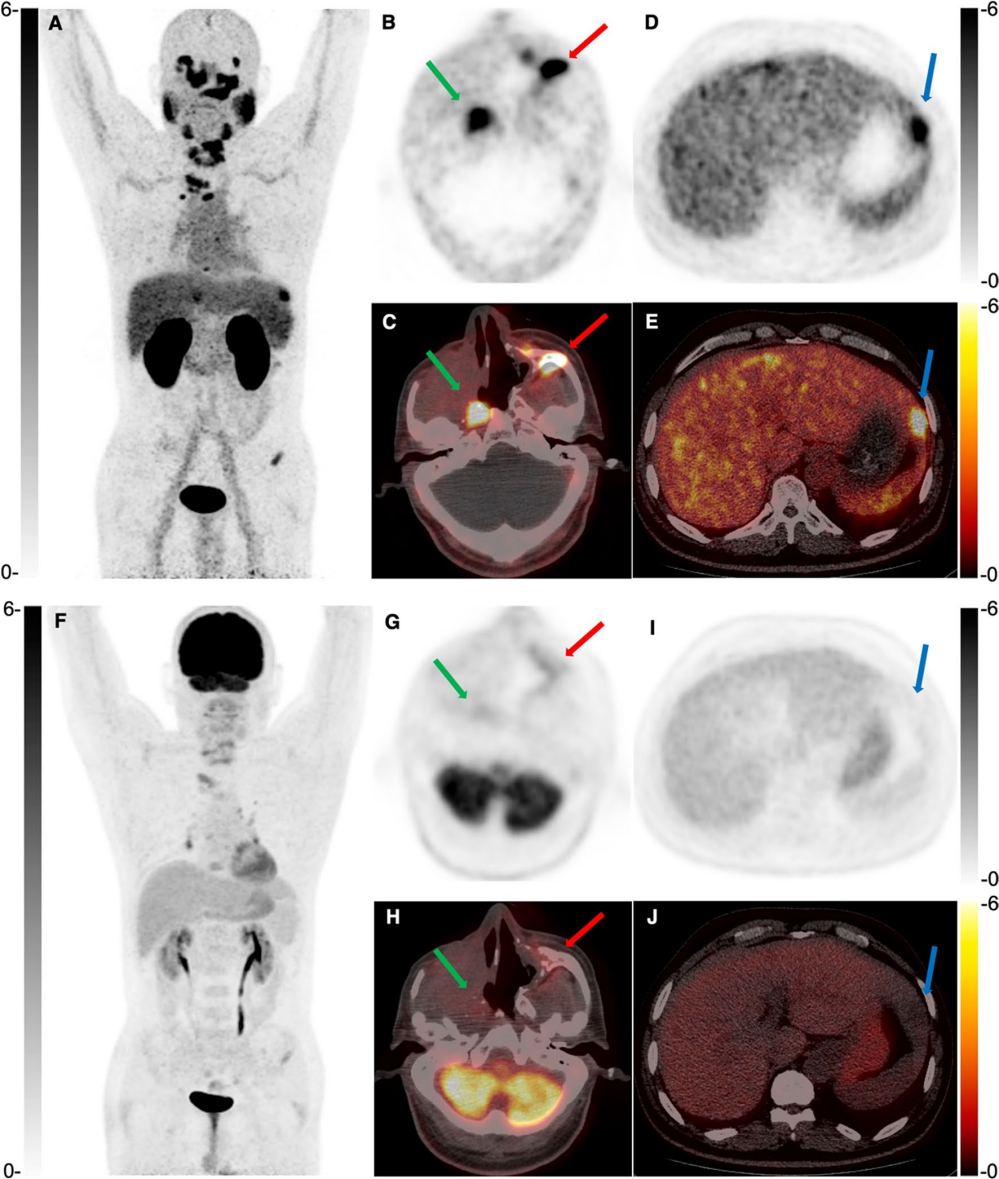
A 62-year-old man was diagnosed with local recurrence and distant metastases 21 months after surgical removal of a right maxillary sinus ACC. ^68^ Ga-PSMA PET/CT (A–E) revealed a PSMA-avid tumor in the right maxillary sinus (green arrow, SUVmax 11.2), multiple bone metastases (red arrow, SUVmax 16.2), and liver metastases (blue arrow, SUVmax 8.8). ^18^F-FDG PET/CT (F–J) showed negative recurrent and metastatic foci
